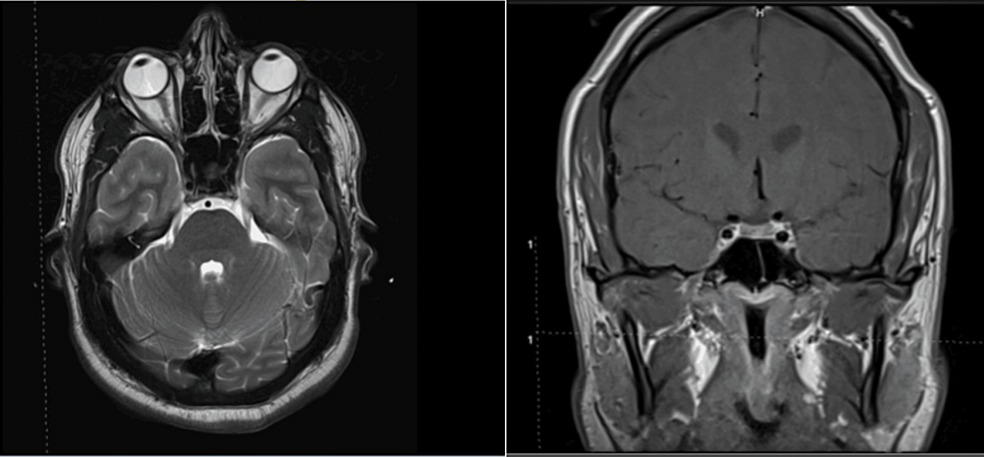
Axial and coronal MR images of the head and orbit showing no abnormalities in the cavernous sinus, orbit, and parasellar regions
